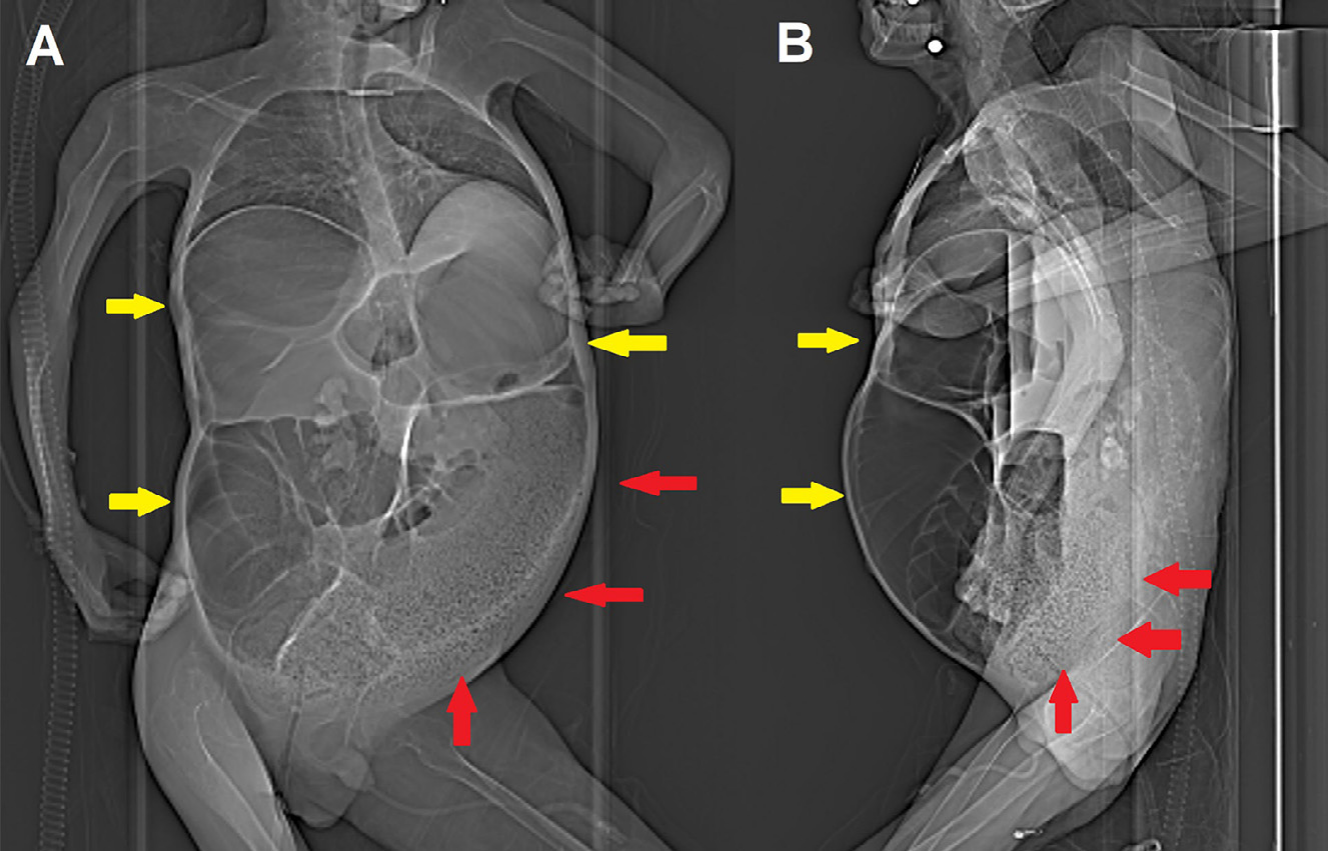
Anteroposterior (A) and lateral (B) scout images obtained before contrast-enhanced CT already show extensive bowel dilatation (yellow arrows) and left colon fecaloma (red arrows).
